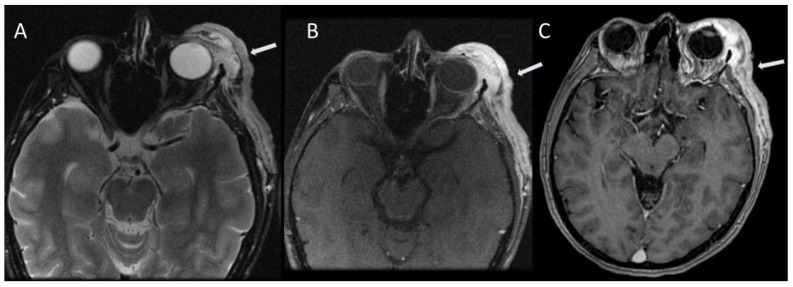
Plexiform neurofibroma (arrows) in the left orbital and temporal region. Axial T2-weighted TSE with fat-suppression (A); axial T1-weighted TSE with fat suppression after gadolinium administration (B); axial T1-weighted TFE after gadolinium administration (C).
